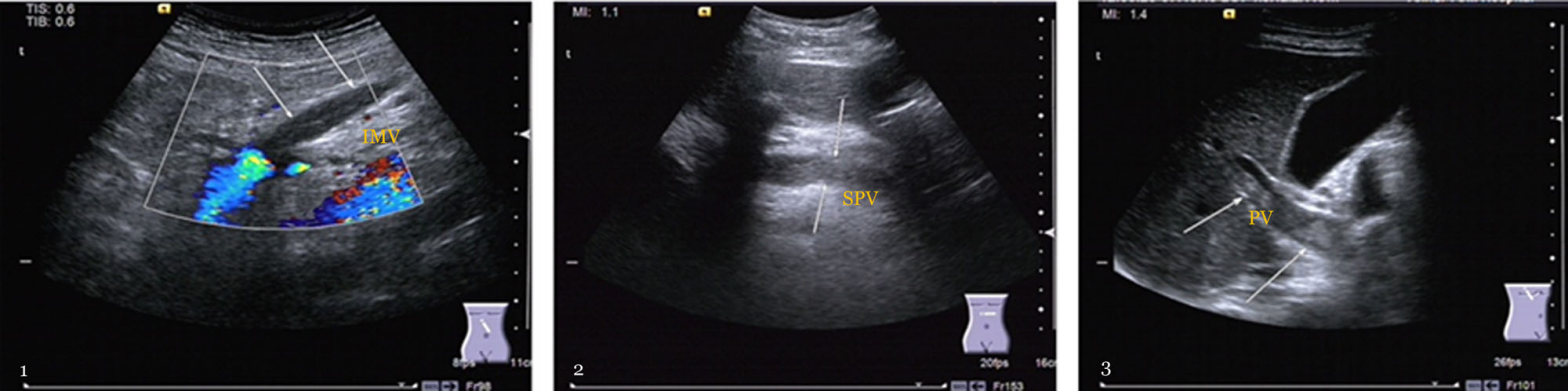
The formation incident of portal vein system thrombosis. 1. Mesenteric vein thrombosis (MVT): gray-scale b-ultrasound image reveals hypoechoic thrombosis within the lumen, and color Doppler ultrasound shows a filling defect in the inferior mesenteric vein (IMV) (arrows). 2. Splenic vein thrombosis: the gray-scale b-ultrasound image shows that splenic vein (SPV) behind the pancreas were filled with hypoechoic thrombus (arrows), and the lumen was blocked. 3. Portal vein thrombosis (PVT): the gray-scale b-ultrasound image demonstrates that the primary portal vein (PV) is closely full of hypoechoic thrombus (arrows), and the main portal vein is almost completely occluded.
